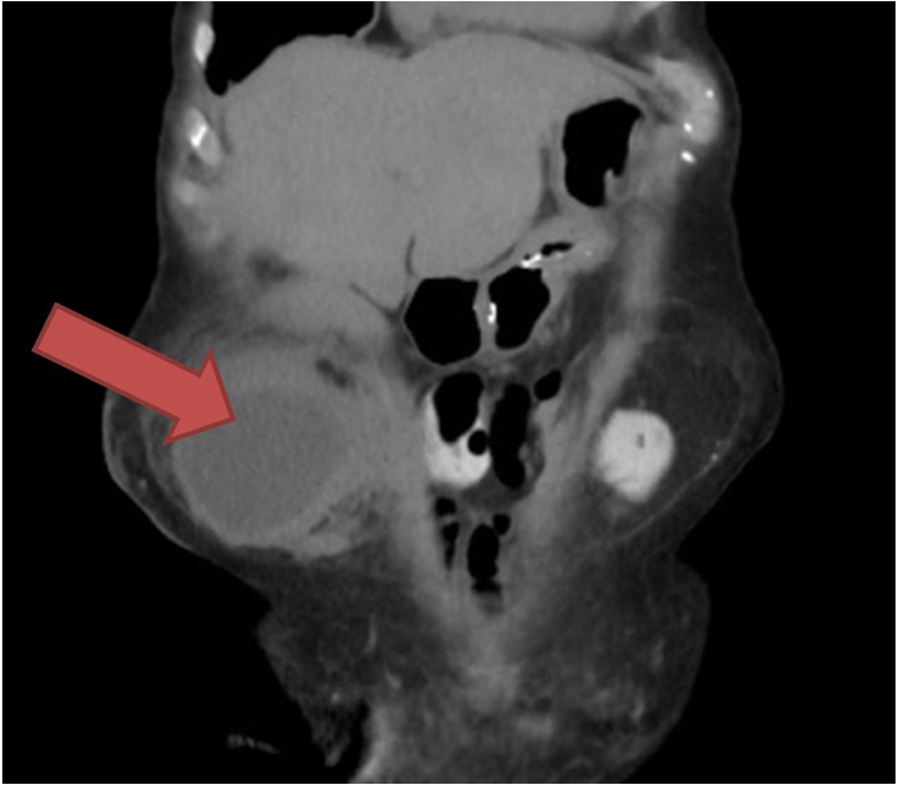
CT appearance in coronal views
